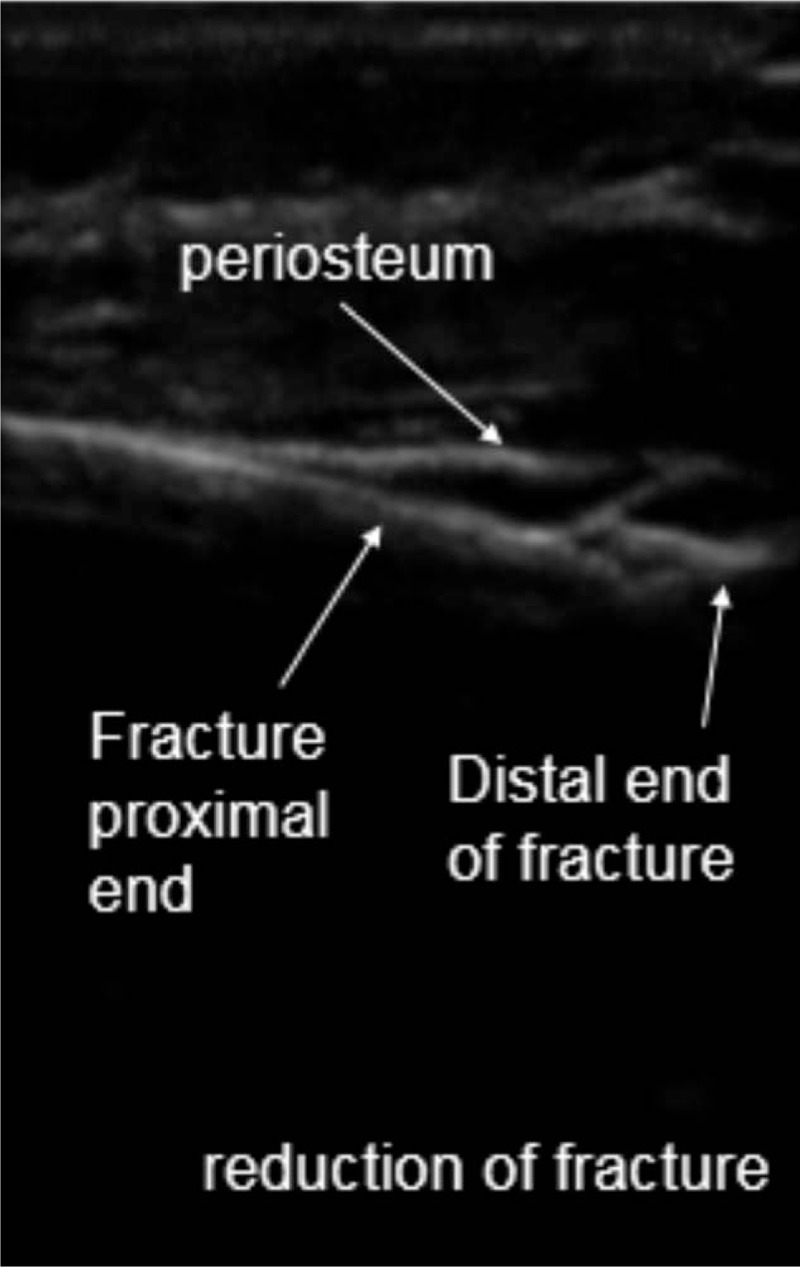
Conducting closed reduction while dynamically monitoring fracture fragments with ultrasound.
